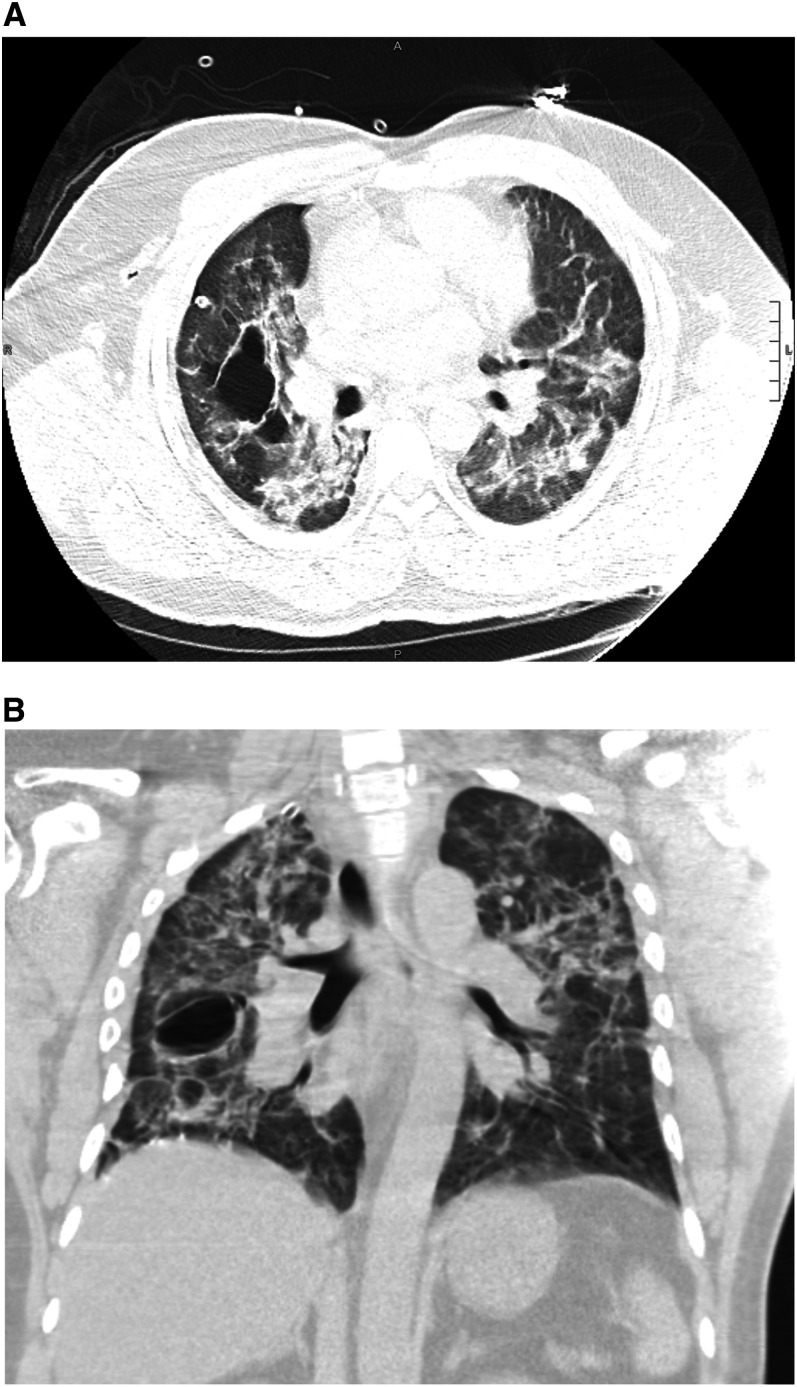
Computed tomography of the chest demonstrating extensive bilateral infiltrates consistent with COVID-19 pneumonia and a right mid-lung bulla measuring 5.6 cm (anteroposteriorly) by 3.3 cm transversely by 2.7 cm craniocaudally. Transverse view (A) and coronal view (B).
